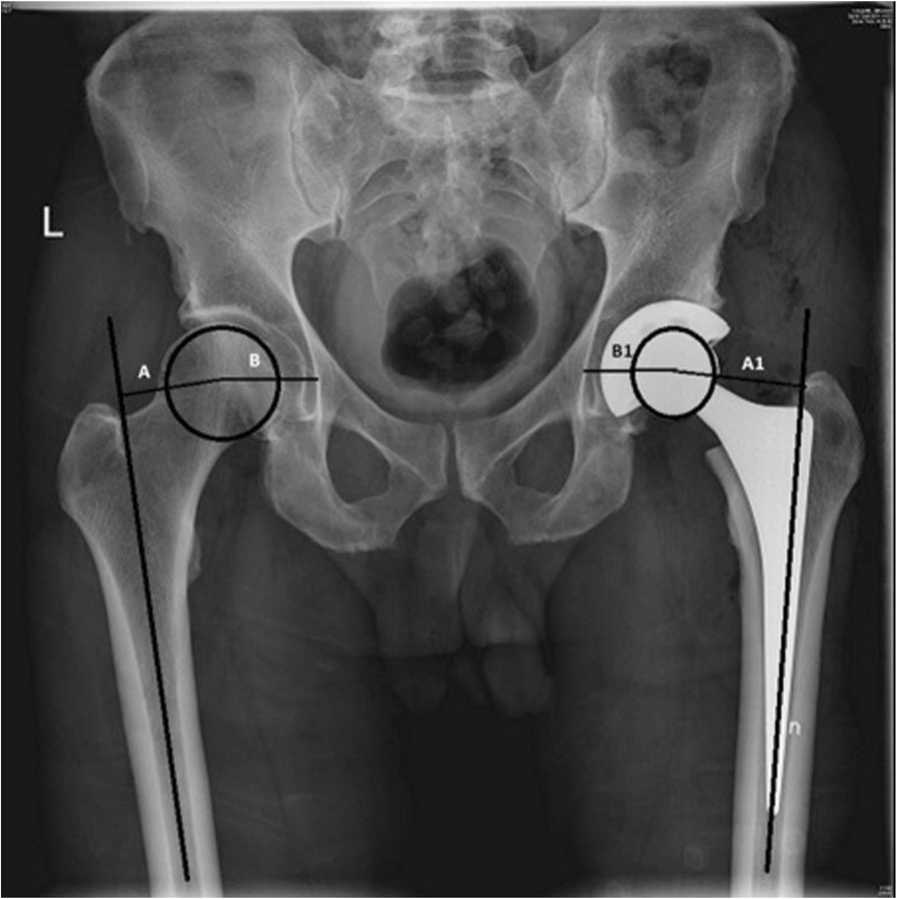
Plain radiograph measurement of the global FO by adding the distance between the longitudinal axis of the femur and the centre of the femoral head (femoral offset) to the distance from the centre of the femoral head to a perpendicular line passing through the medial edge of the ipsilateral teardrop point of the pelvis (cup offset)
